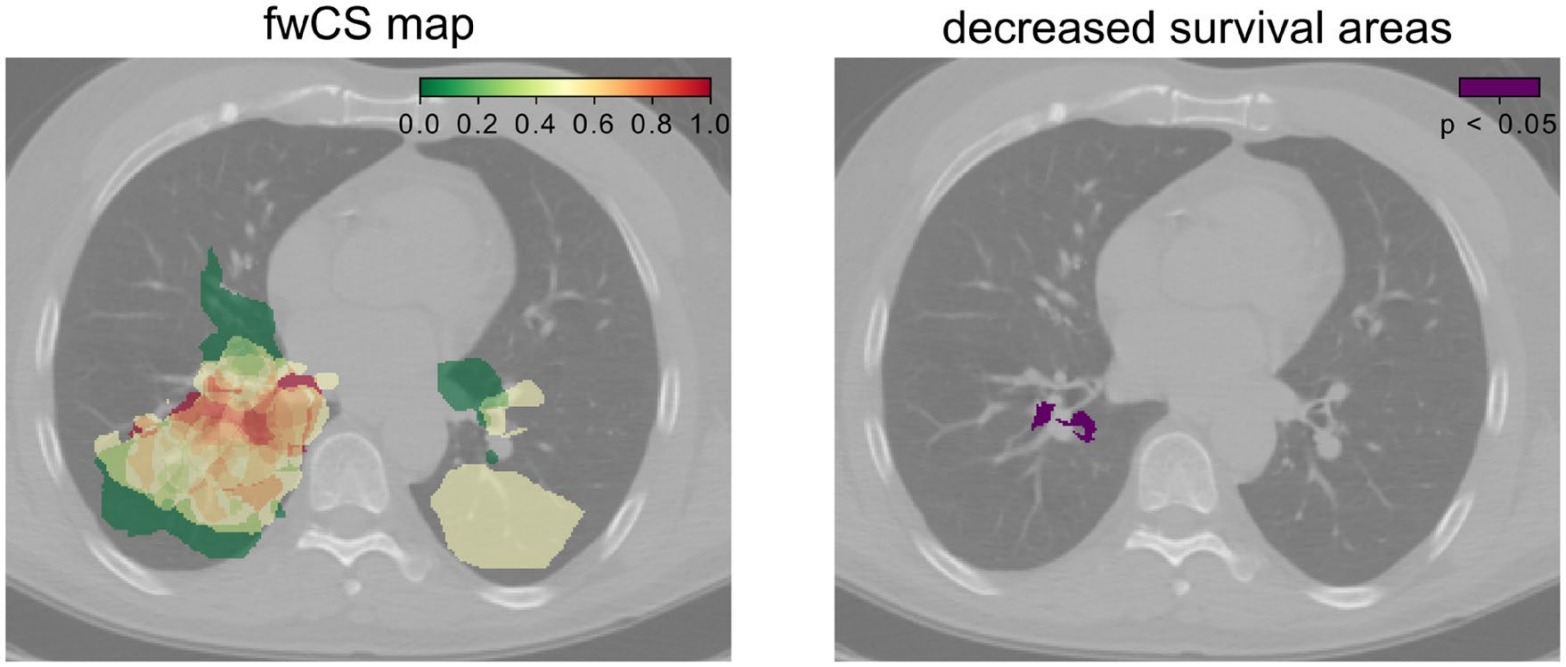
Axial CT slice of S1 frequency weighted cumulative status (fwCS) map on the left and decreased survival areas (DSA) labeled using the permutation method. Violet areas indicate statistically significant regions. Significant areas were found in the right lung close to the mediastinum.
